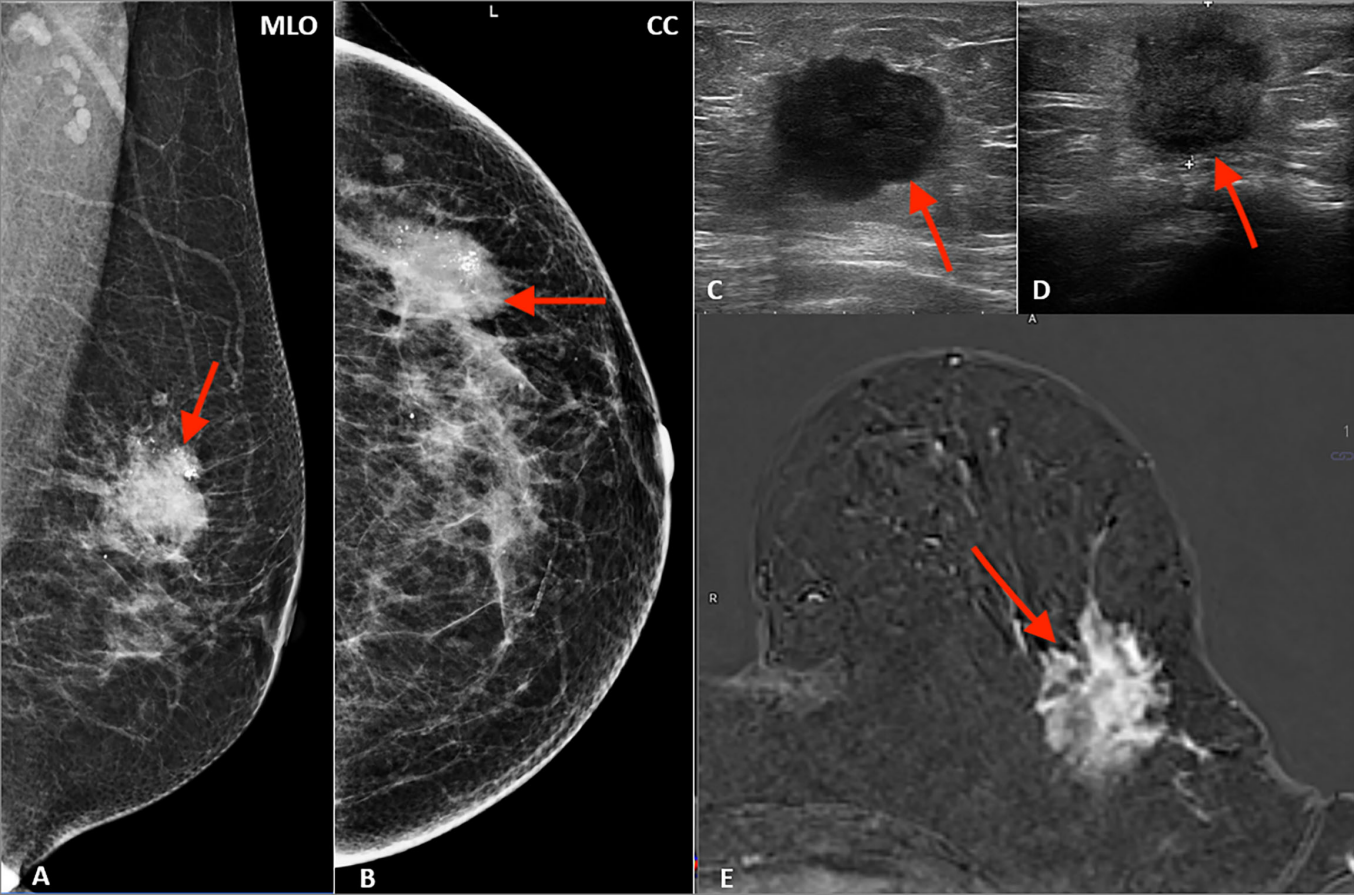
Multimodality presentation of lobular breast cancer; Patient 1 left breast: (A, B) mammography: MLO (A) and CC (B)—not circumscribed, spiculated mass with microcalcifications (red arrow); (C, D) ultrasound—not circumscribed, spiculated, hypoechoic mass (red arrow); (E) MRI T1 post contrast—not circumscribed, spiculated mass with heterogenous contrast enhancement (red arrow).
